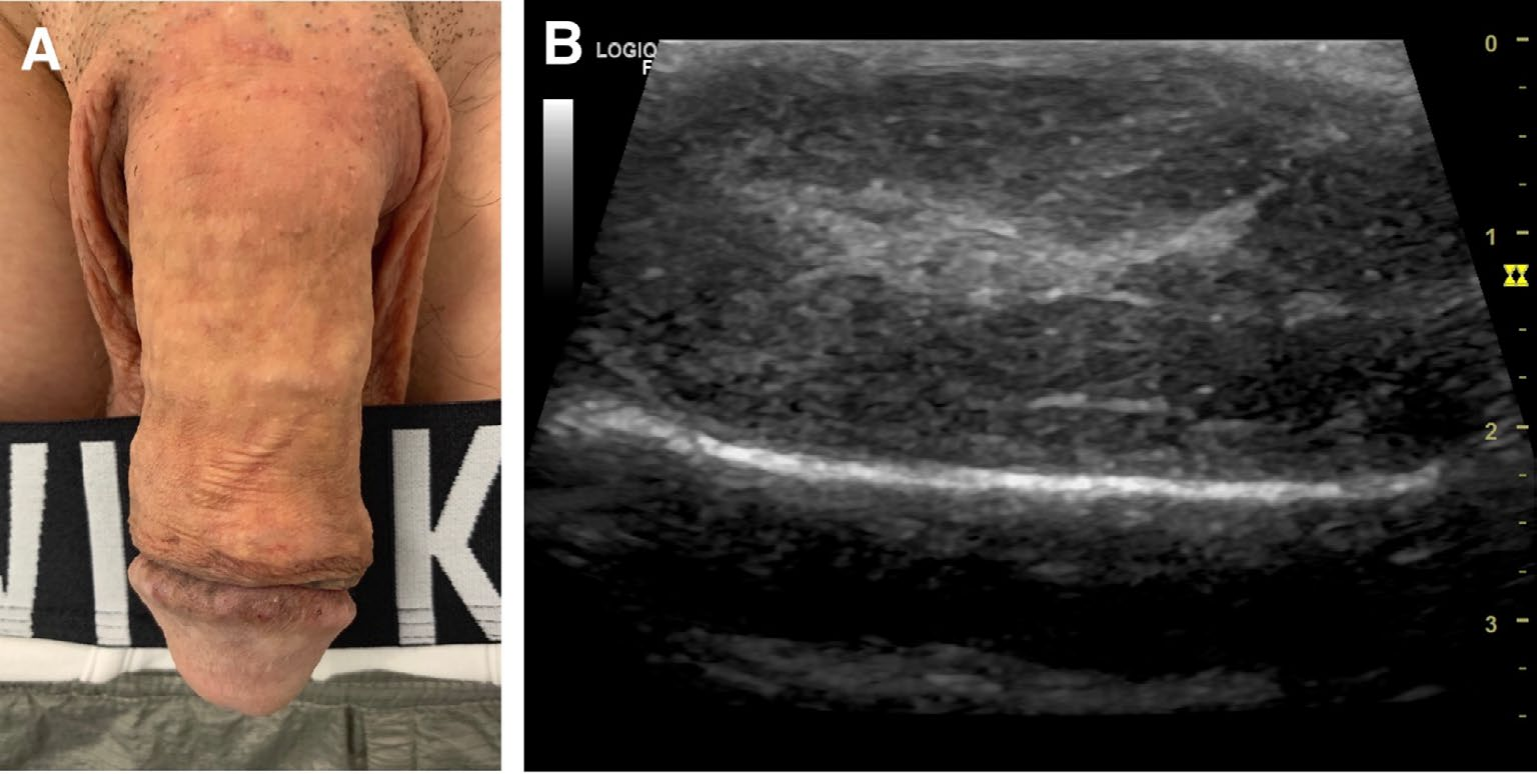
Third case. (A) diffuse subcutaneous induration of the penis, (B) ultrasound image.
